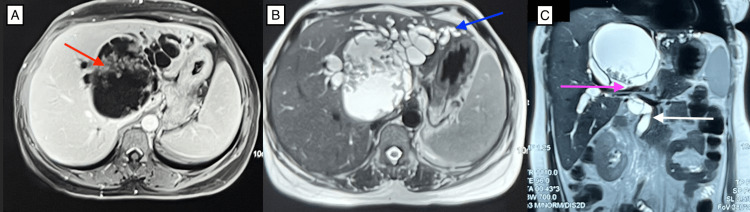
MRI abdomen (A) MRI abdomen showing T1 hypointense cystic lesion with papillary excrescences (red arrow). (B) The lesion is hyperintense in the T2 phase with dilatation of the left biliary system (blue arrow). (C) The cyst is compressing the confluence of the right and left hepatic ducts (pink arrow). The dilated common bile duct can also be appreciated (white arrow).
